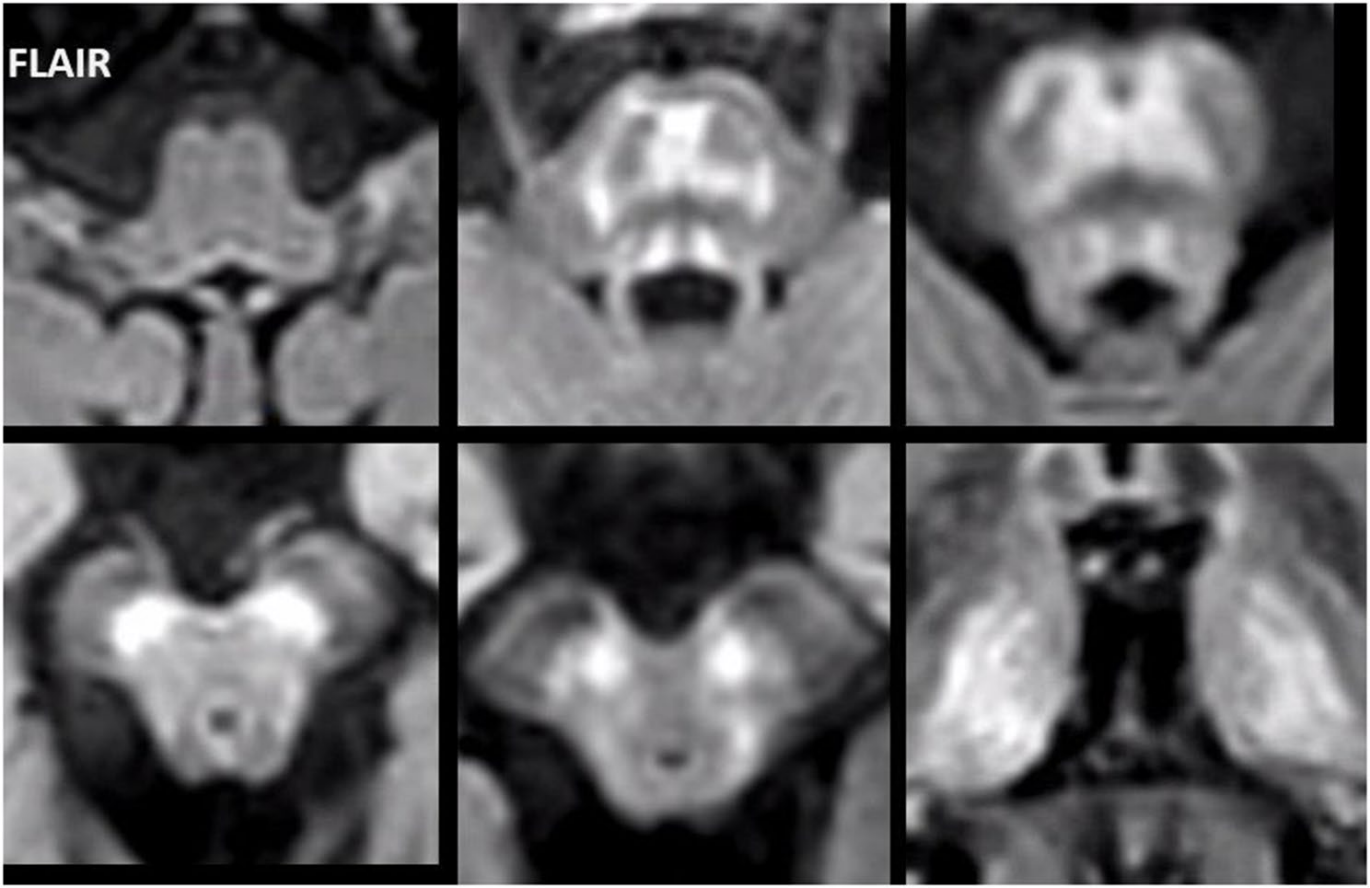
FLAIR images of patient 4 show a normal appearance of the medulla oblongata. The signal abnormalities of the pons are continuous with the affected substantia nigra. The red nucleus and thalamus are also symmetrically affected
